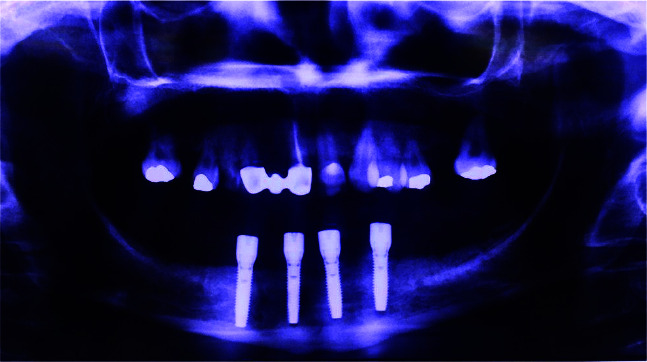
Panoramic radiograph immediately after implant surgery
